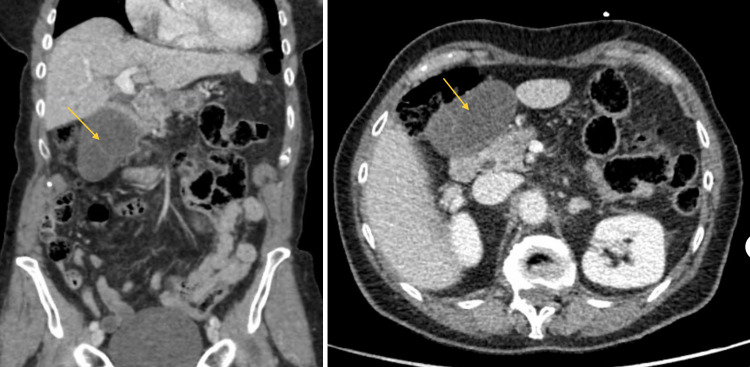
CT abdomen/pelvis. CT abdomen/pelvis revealed a dilated common bile duct and central biliary tree. The gallbladder was found to be dilated, redundant, and exhibited heterogeneous density possibly related to sludge. Gold arrows indicate the gallbladder.
